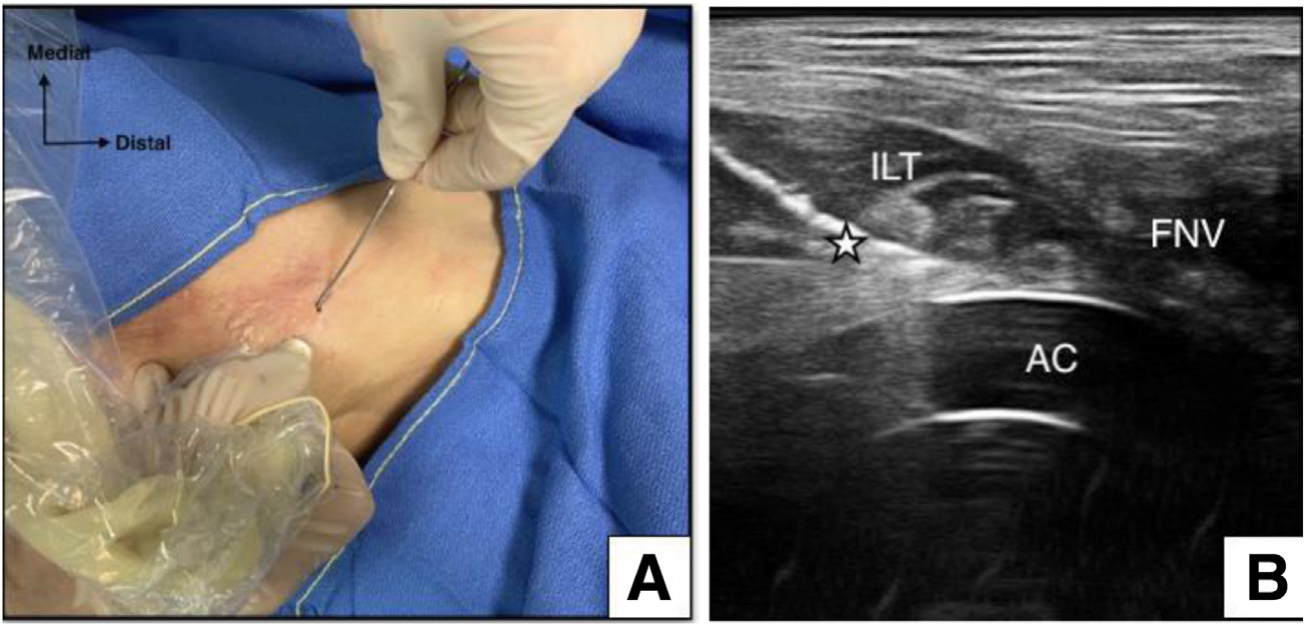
(A) Top view of hooked blade introduction guided from lateral to medial to iliopsoas tendon under ultrasound-guided control with positioning orthogonal to its fibers lying over acetabular cup. (B) Right hip in supine cadaveric model. The star indicates tenotomy of the fibers. (AC, acetabular cup; FNV, femoral neurovascular bundle; ILT, iliopsoas tendon.)
